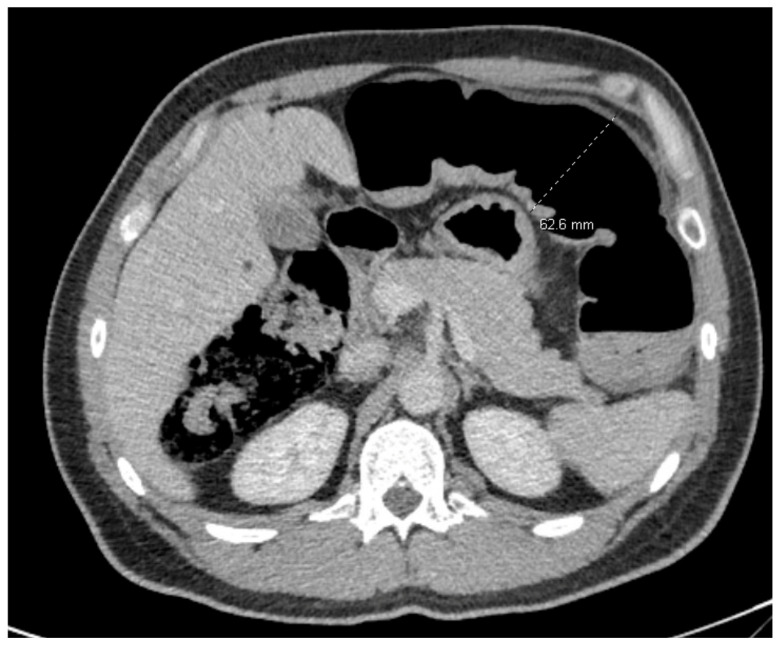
Axial contrast-enhanced CT of the abdomen demonstrating marked proximal colonic dilatation, measuring approximately 6.3 cm, secondary to a diverticulitis-related colonic stricture causing mechanical large-bowel obstruction.
